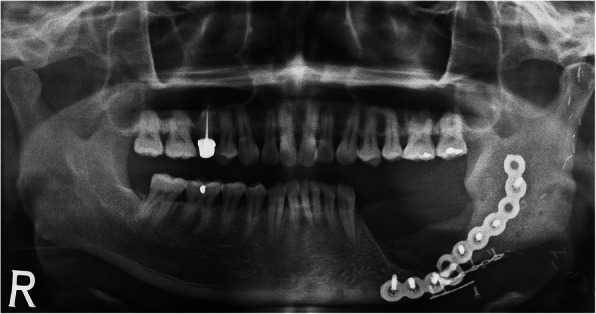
Pathologic fracture of the left mandibular body was observed in the panoramic radiograph
